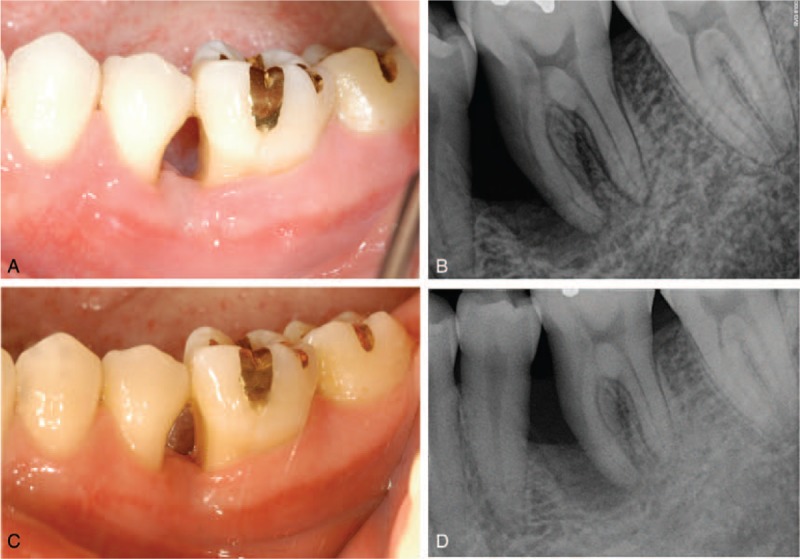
Postoperative follow-up. (A) Uneventful healing was achieved and sutures were removed 2 weeks after the operation. (B) Two-week postoperative periapical radiograph. (C) Two-month postoperative clinical photograph without symptoms. (D) Two-month postoperative periapical radiograph.
